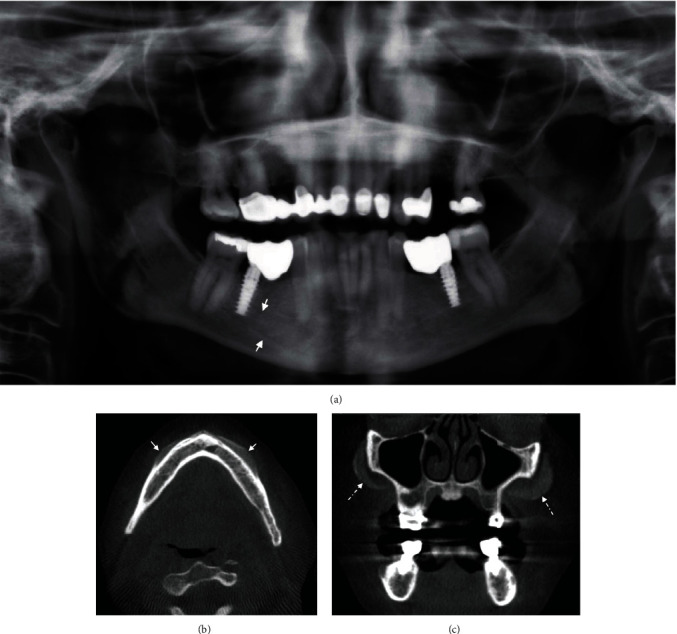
Subperiosteal cosmetic filler in the mandible (case 1): (a) panoramic radiograph showing linear radiopaque lines (arrows); (b) axial CBCT image showing a bilateral, subperiosteal cosmetic filler at the buccal cortex of the posterior mandible; (c) coronal CBCT image showing bilateral zygomatic implants (dotted arrows).
